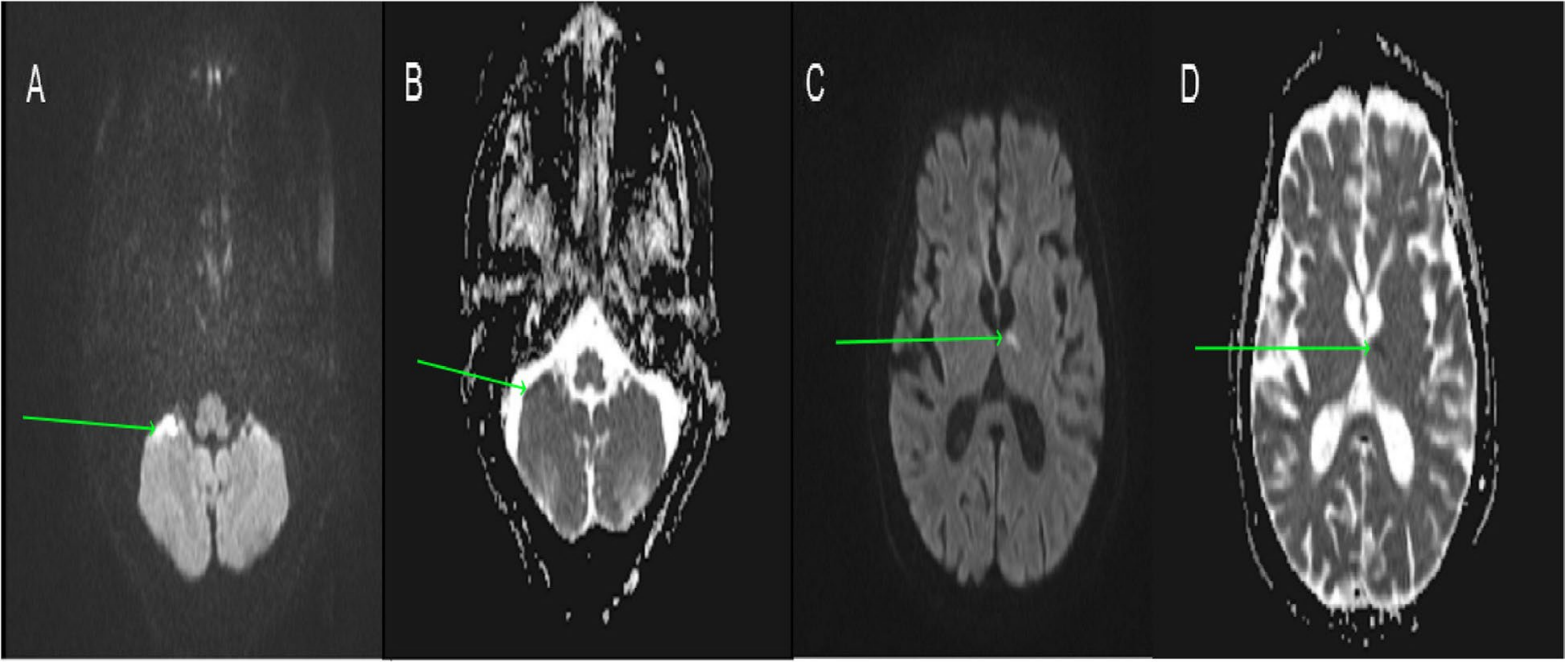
A Axial section. Magnetic resonance imaging of the brain showing diffusion restriction in right cerebellar hemisphere (green arrow). B Axial section magnetic resonance imaging of the brain showing low apparent diffusion coefficient values in right cerebellar hemisphere (green arrow). C Axial section magnetic resonance imaging of the brain showing diffusion restriction in left thalamus (green arrow). D Axial section magnetic resonance imaging of the brain showing low apparent diffusion coefficient values in left thalamus (green arrow)
